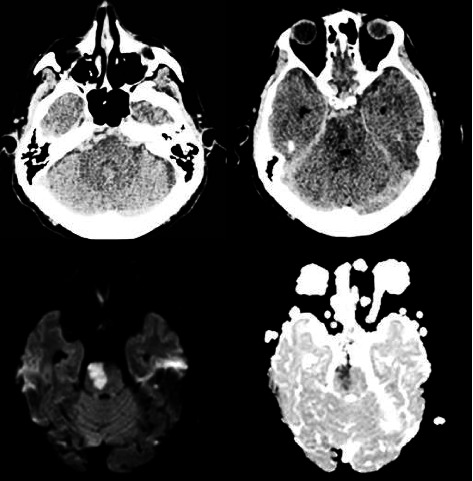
Admission brain CT-Day 3 and MRI Day 4 after symptom onset showing pretruncal perimesencephalic subarachnoid hemorrhage and paramedian pontine infarction.
